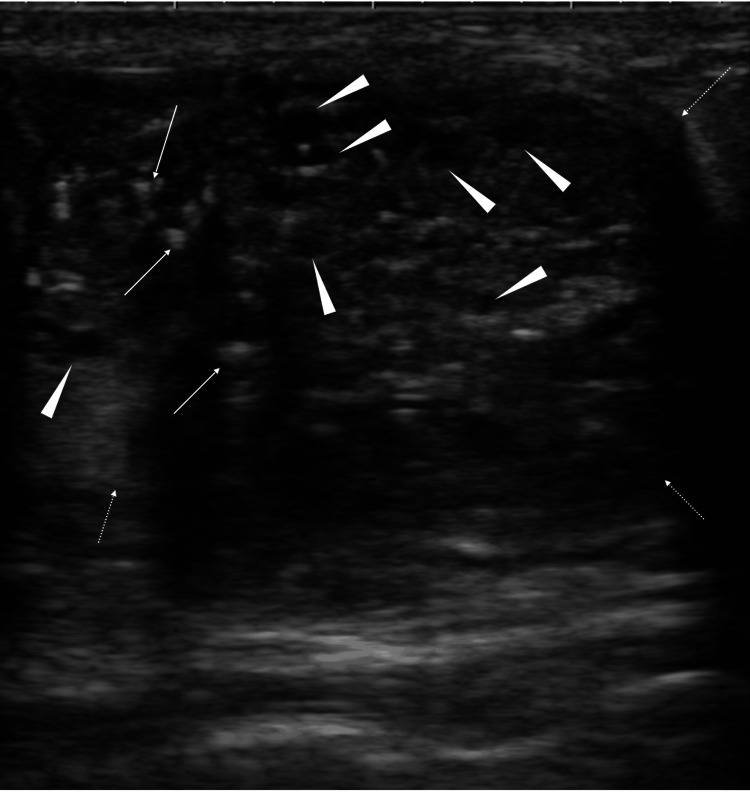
Ultrasound findings Ultrasound showed a well-circumscribed mass (white dotted arrows) with multiple echogenic spots (white arrows) presumably due to the presence of micro calcifications and many small cysts (white arrowheads).
